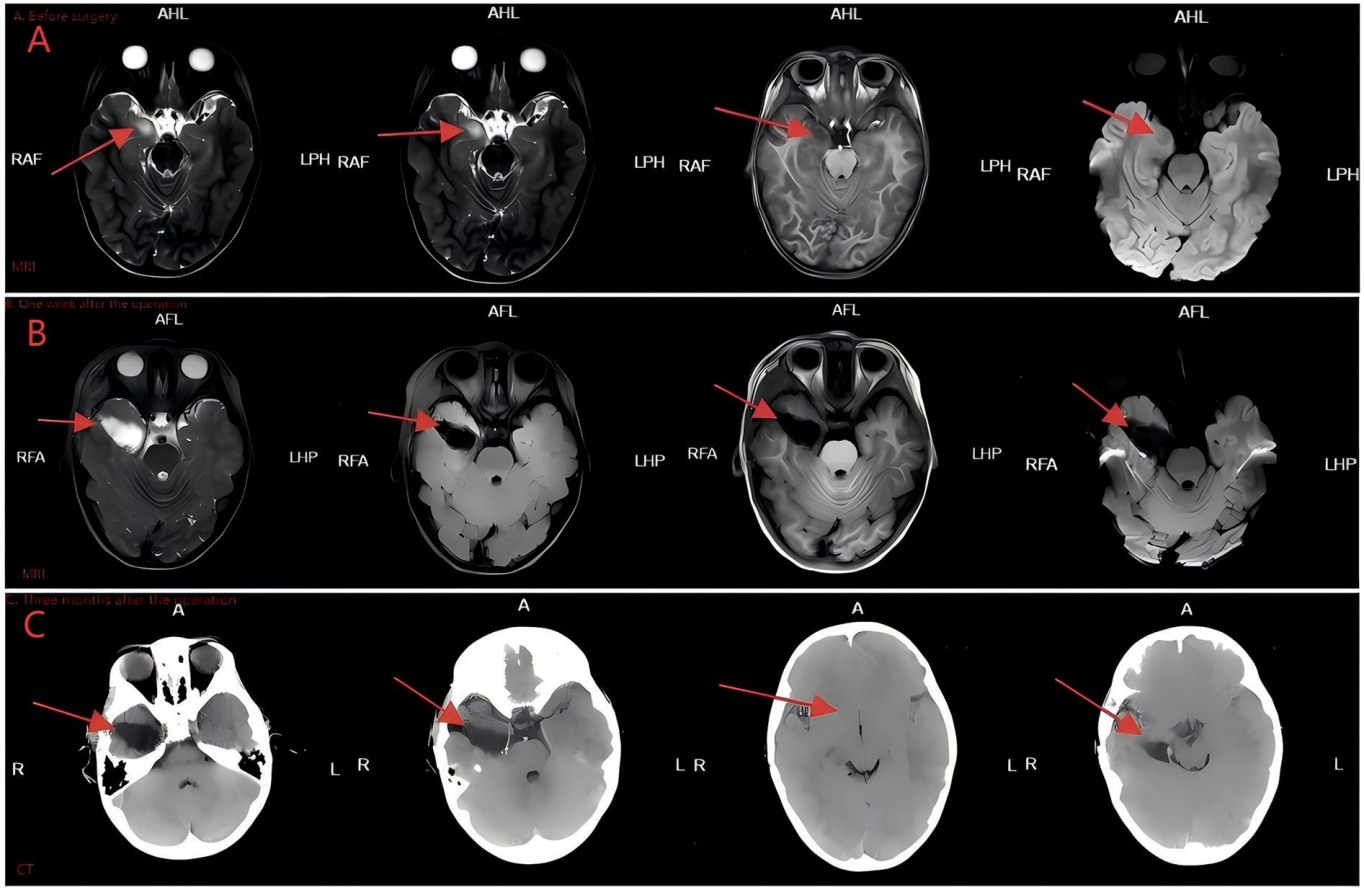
Head imaging examination. (A) Preoperative cranial MRI. (B) Cranial MRI obtained one week postoperatively. (C) Cranial CT scan obtained three months postoperatively.
